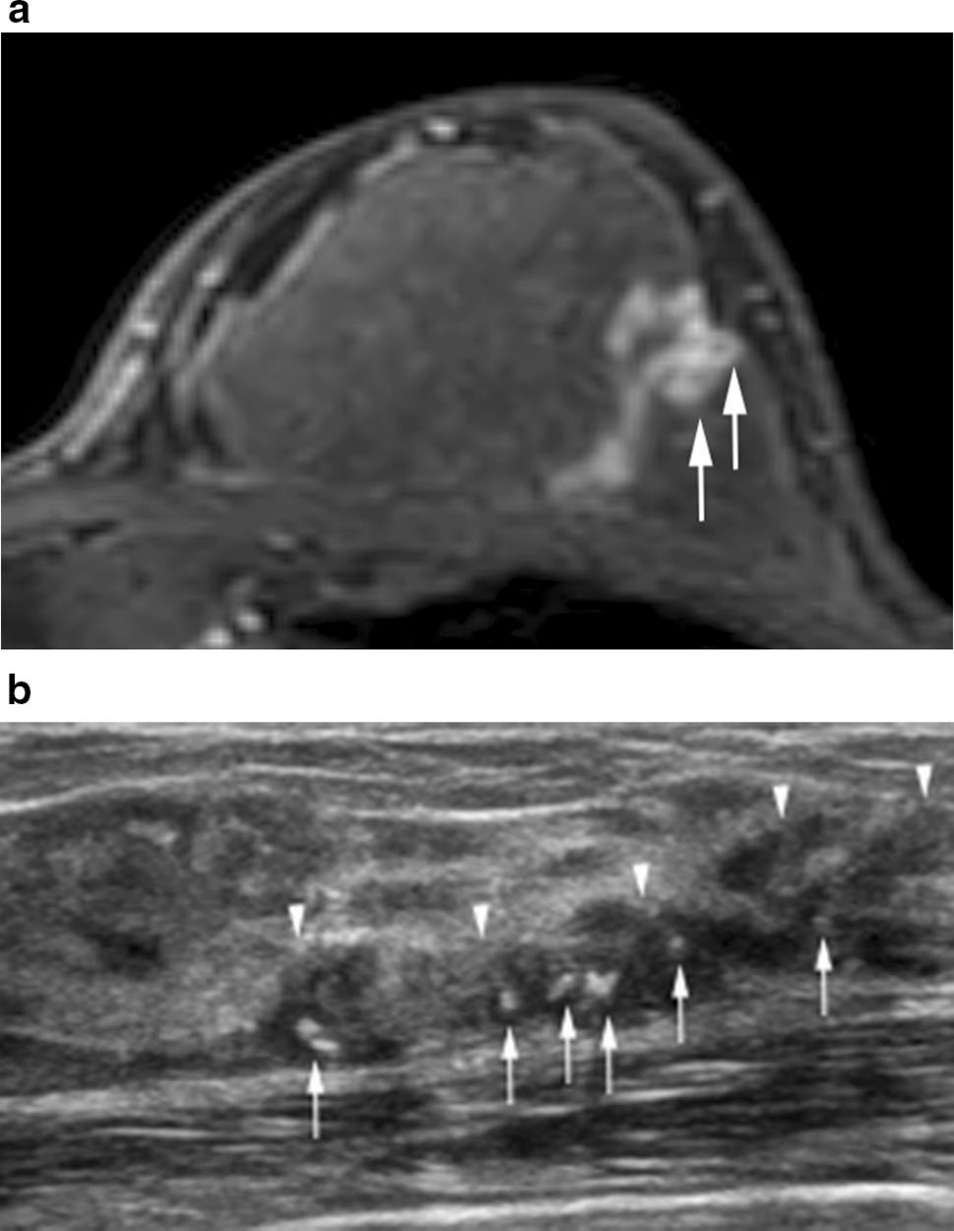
High-grade DCIS in the left upper outer breast in a 61-year-old woman. a The axial postcontrast T1-weighted image shows clumped linear enhancement with clustered ring enhancements (arrows). b The corresponding US image shows a clumped linear non-mass lesion (arrowheads) with associated calcifications (arrows)
